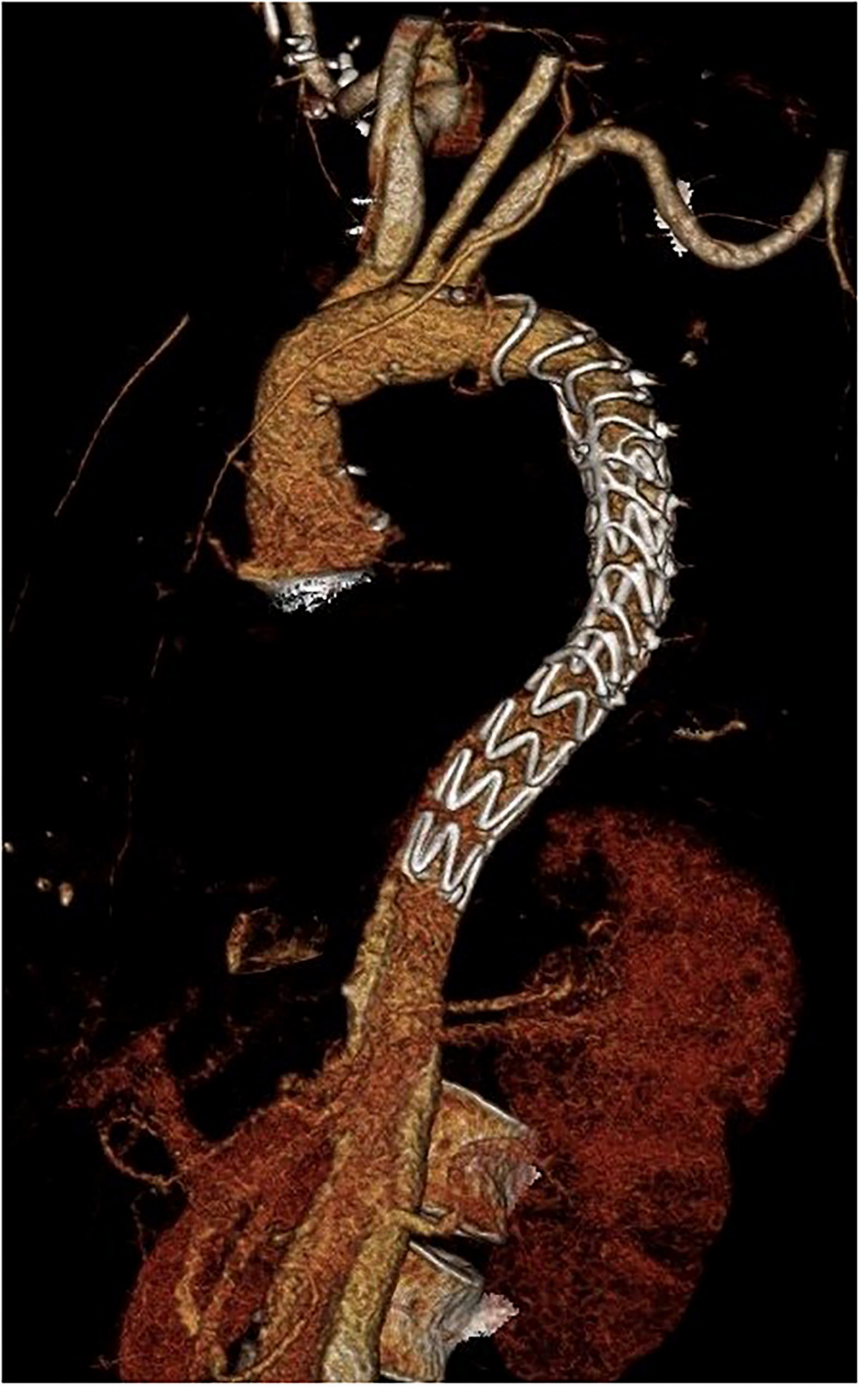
Three-dimensional computer tomography angiography (CTA) after distal stentgraft extension succeeding after implantation of the Thoraflex Hybrid prosthesis.
